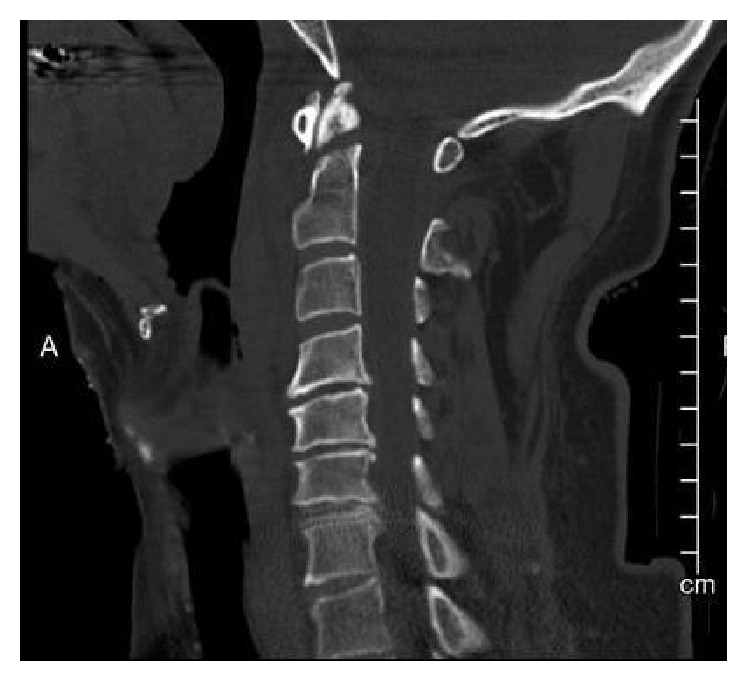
Midaxial sagittal cervical spine CT showing a slightly distracted Type II dens fracture without additional cervical spine fracture.
